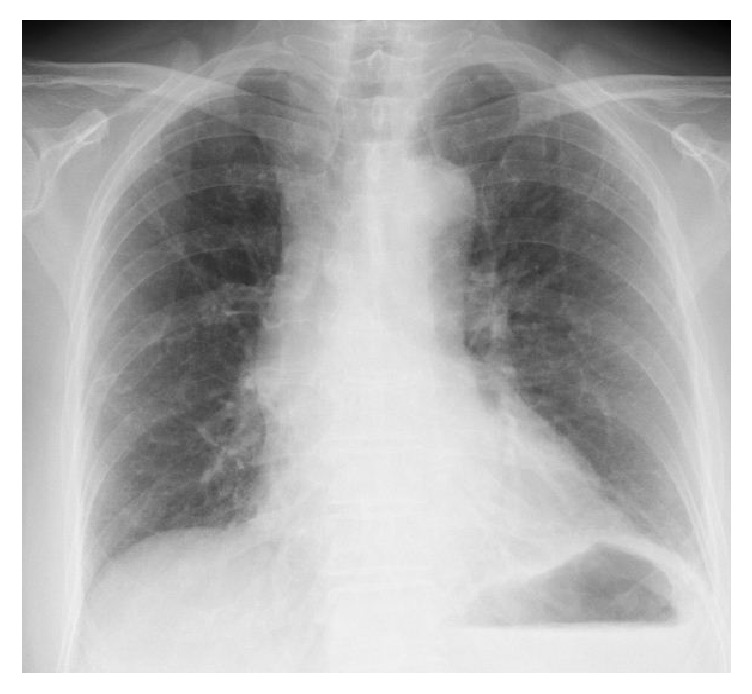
Chest X-ray one and a half months after the initiation of everolimus treatment, showing no infiltrative shadows in both lung fields.
